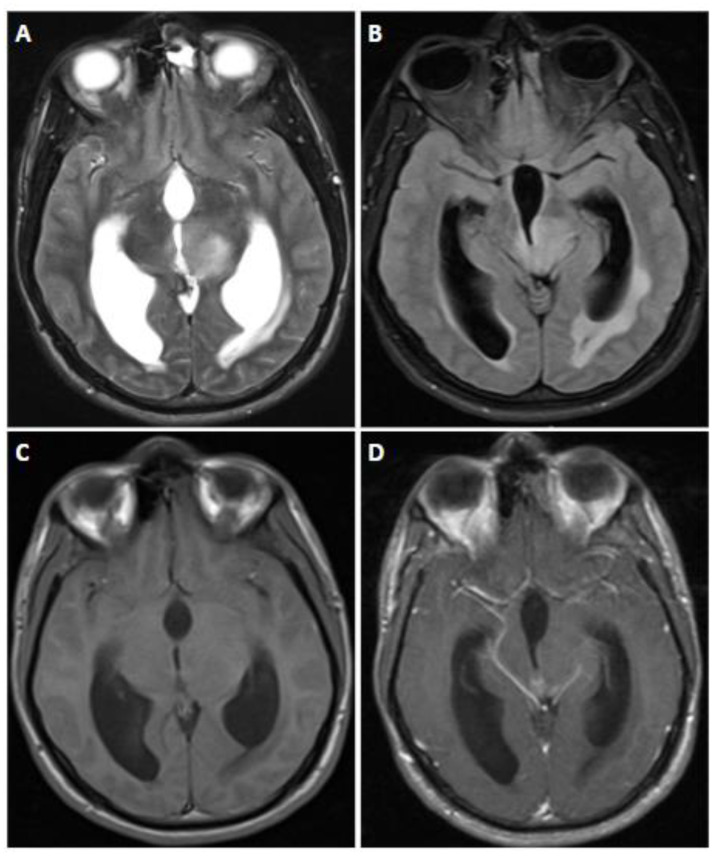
Axial T2-weighted (A), axial T2 fluid-attenuated inversion recovery (FLAIR) (B), axial T1-weighted (C), and axial T1-weighted post-contrast (D) images in 27 year old man with high-grade astrocytoma with piloid features (HGAP). Images demonstrate a heterogeneous T2 hyperintense mass centered in the left thalamus, extending into the tectal plate. There were some areas of heterogeneous enhancement. Local mass effect and obstruction at the level of the cerebral aqueduct resulted in upstream obstructive hydrocephalus. Molecular profiling showed isocitrate dehydrogenase (IDH) wildtype, alpha-thalassemia/mental retardation, X-linked (ATRX), Neurofibromin 1 (NF1) mutation, and cyclin-dependent kinase inhibitor 2A/B (CDKN2A/B) homozygous deletion. Collective molecular features suggest high-grade astrocytoma with piloid features (HGAP), according to WHO CNS5 tumor classification.
